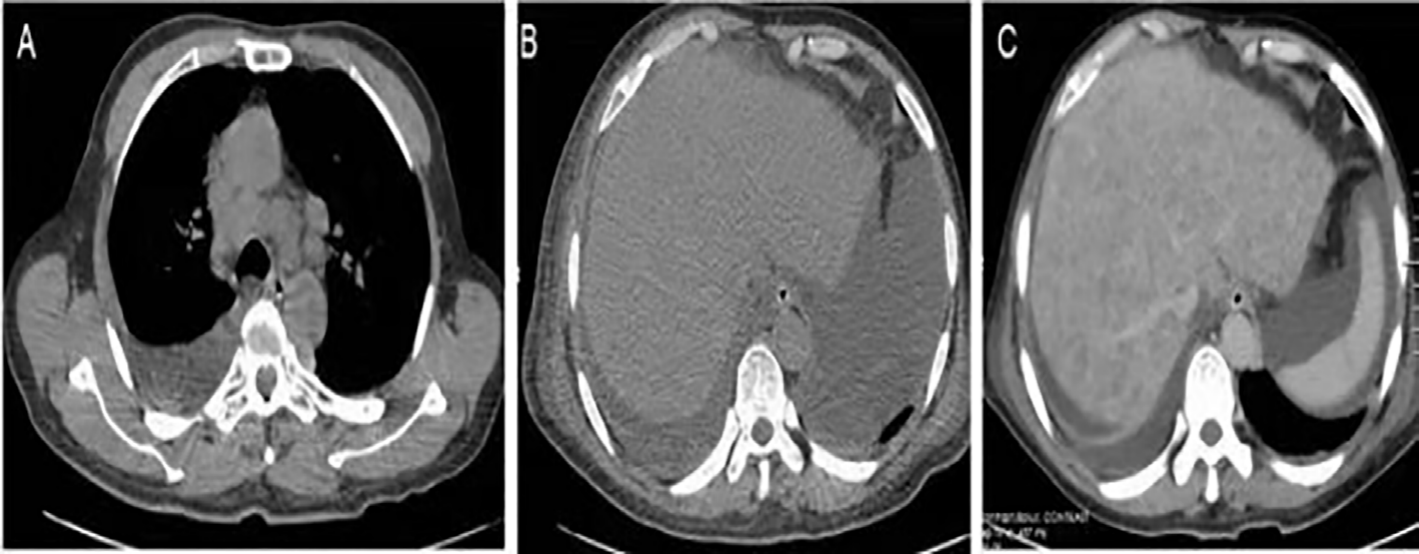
Imaging findings. (A) Chest CT revealed enlarged lymph nodes in the mediastinum. (B) Abdominal CT showed ascites with no hepatic focal lesions. (C) Contrast-enhanced CT of the abdomen revealed diffuse regenerative nodules, cholecystitis, peritonitis, and ascites. No splenomegaly, biliary obstruction, or pulmonary nodules were observed.
